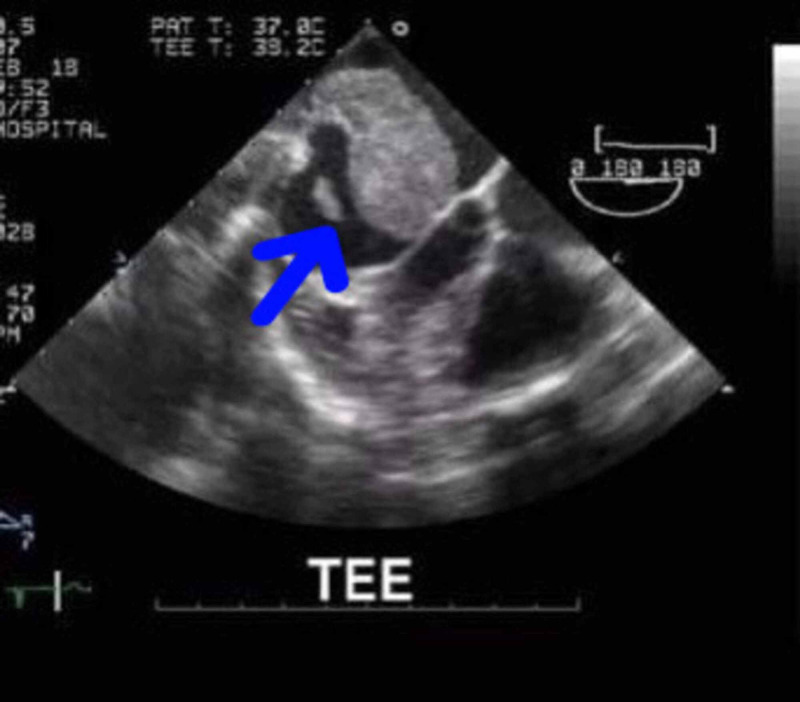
TEE before surgery TEE, transesophageal echocardiogram
